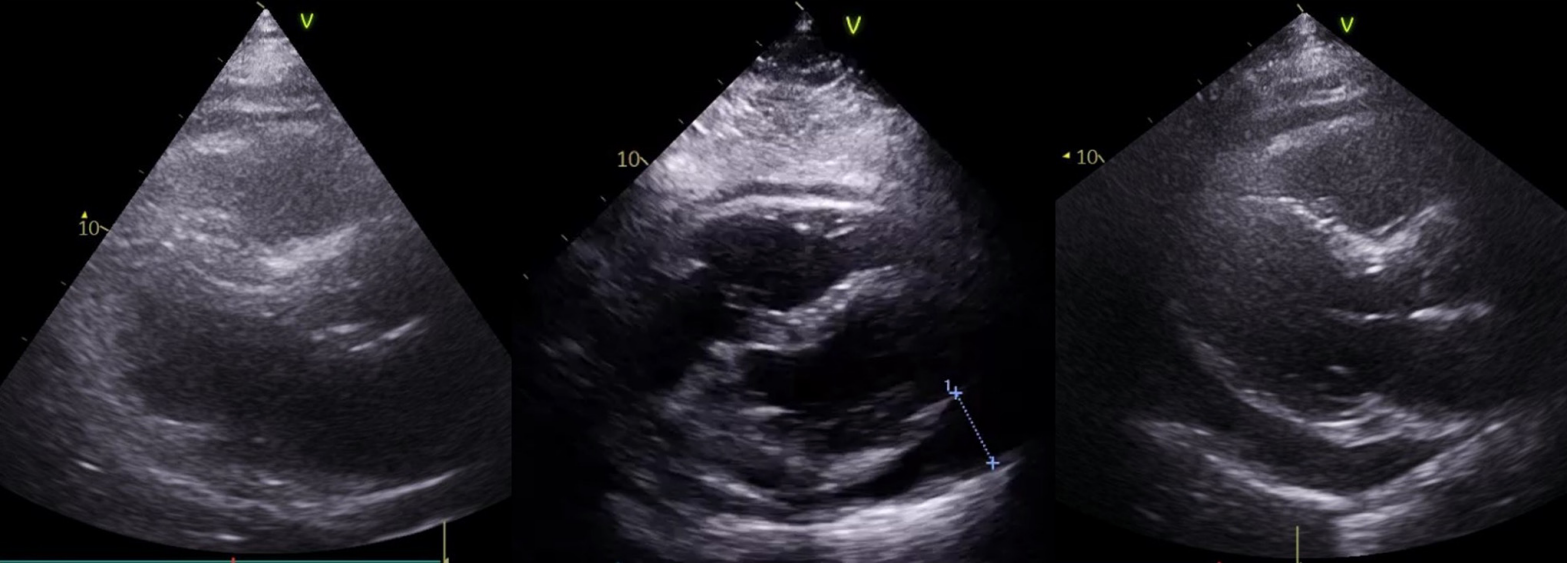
Transthoracic echocardiographic images showing progression of pericardial effusion. The leftmost image is a parasternal long-axis view at day 4. The middle image is a subcostal view at day 8. The rightmost image is a parasternal long-axis view at day 14 with circumferential pericardial effusion measured at a maximum of 2.6 cm. No significant pericardial fat was demonstrated on these echocardiographic images.
